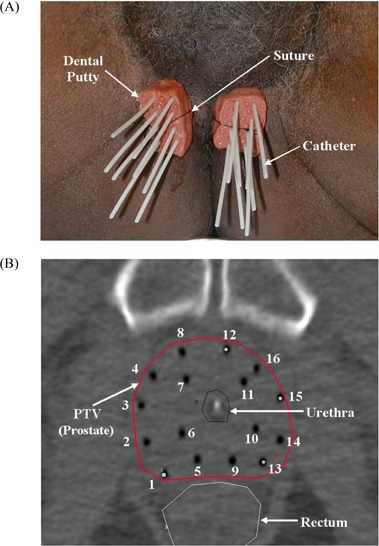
(A) Fixation of 16 catheters on the perineum using dental putty. (B) Typical pattern of 16 catheters inserted into the prostate (axial computed tomography slice for mid‐gland of prostate).
